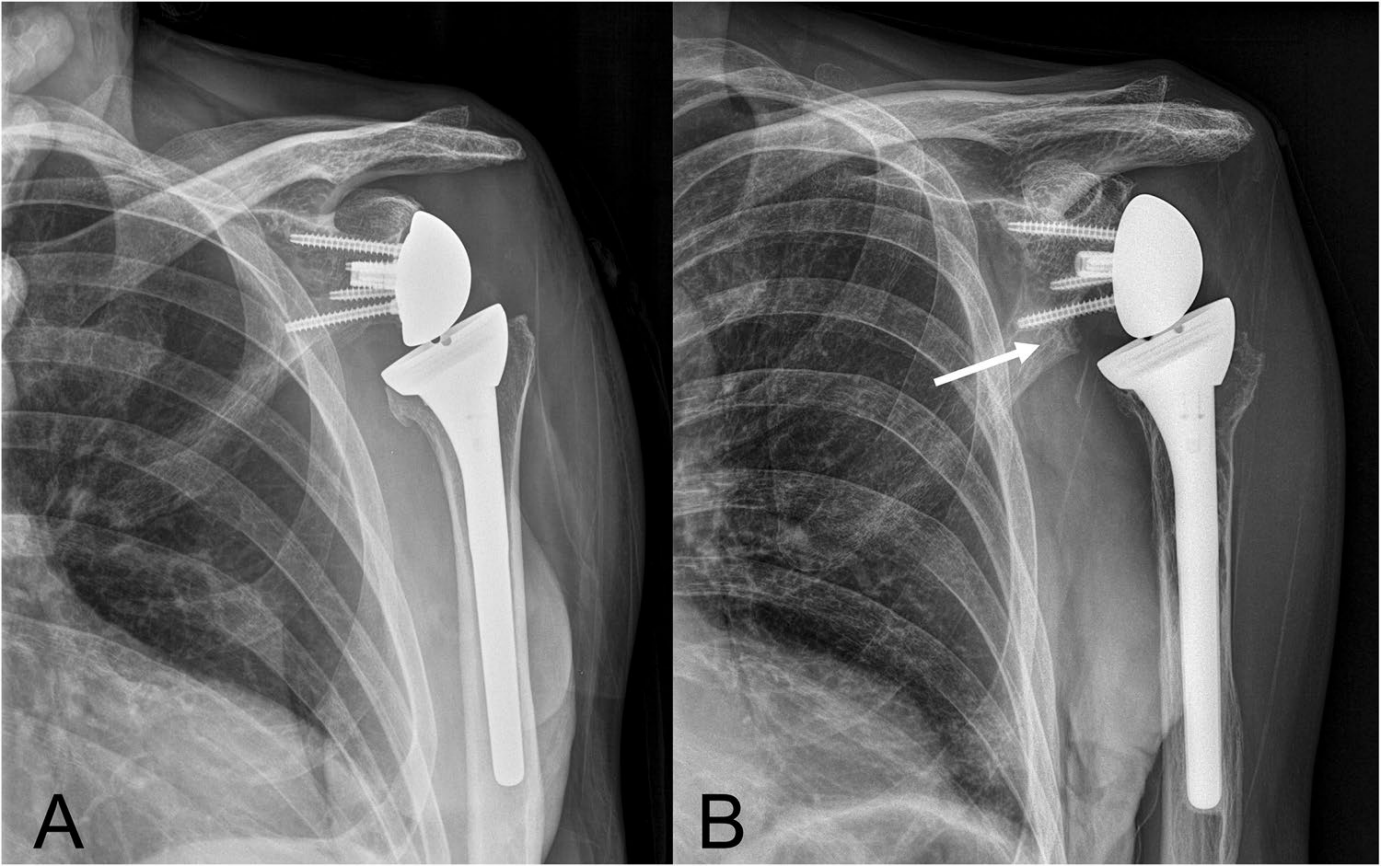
Demonstrates a Grammont design immediately postoperatively (A) as well as an 8-year follow-up radiograph where scapular notching is demonstrated (white arrow) (B)
